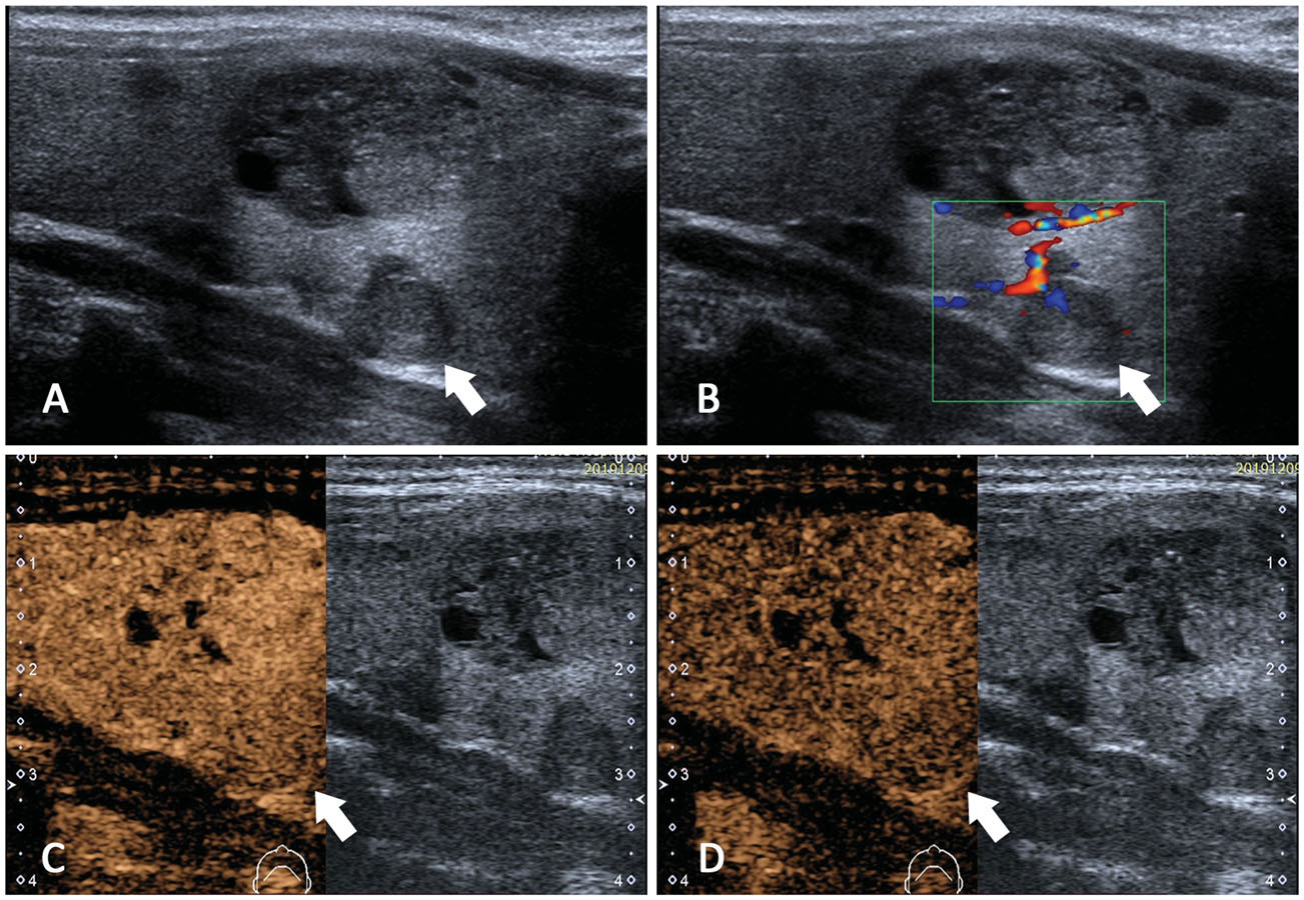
Ultrasonography feature of SHPT patient with low iPHT. A 52-year-old male diagnosed as SHPT for 4 years with low iPTH(443.5pg/ml). (A) CUS detects a hypoecho lesion about 1.0 cm (white arrow). (B) Color Doppler showed the lesion has grade I blood supply. (C,D) In CEUS, this lesion appeared to be iso-enhanced in both early and late phase.
